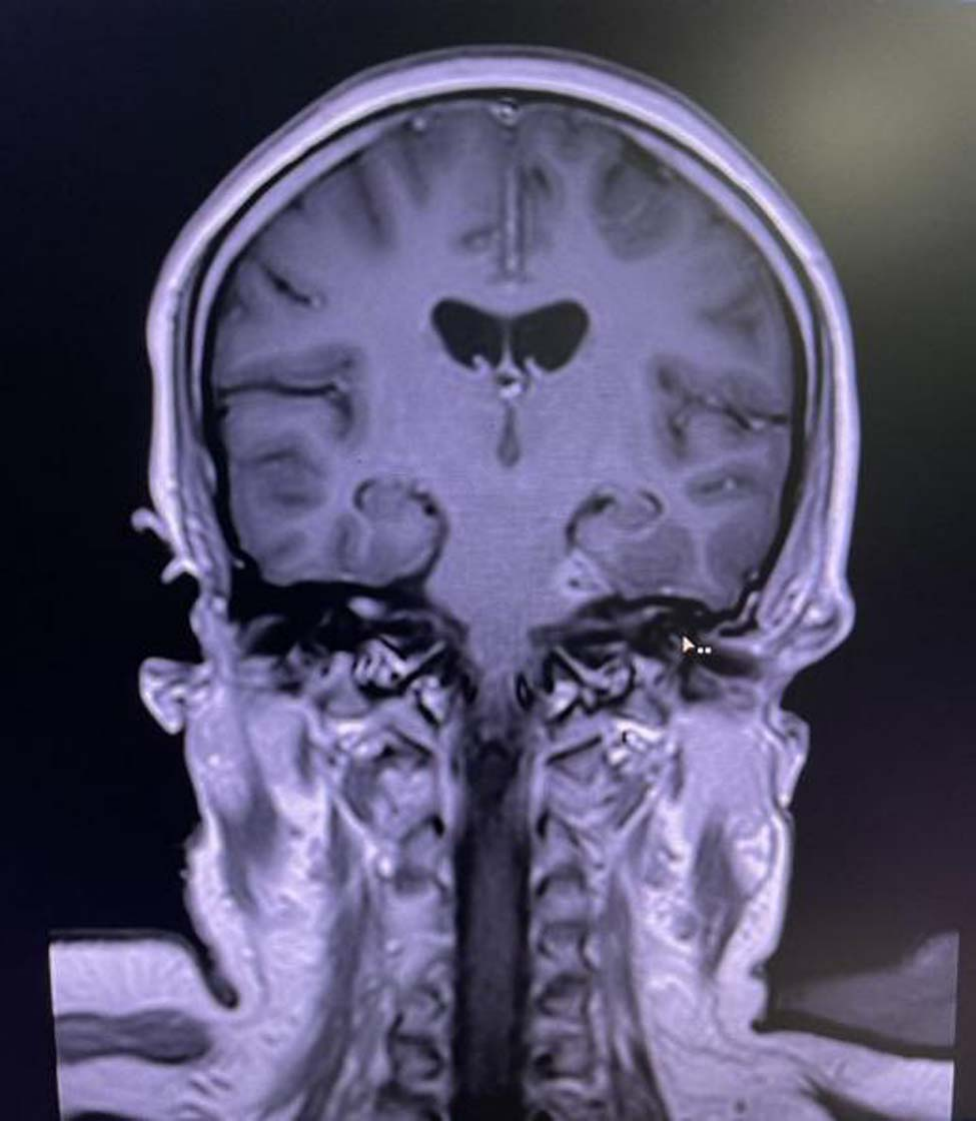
Coronal MRI showing enhancement in the left cerebellopontine angle along the trigeminal nerve.
